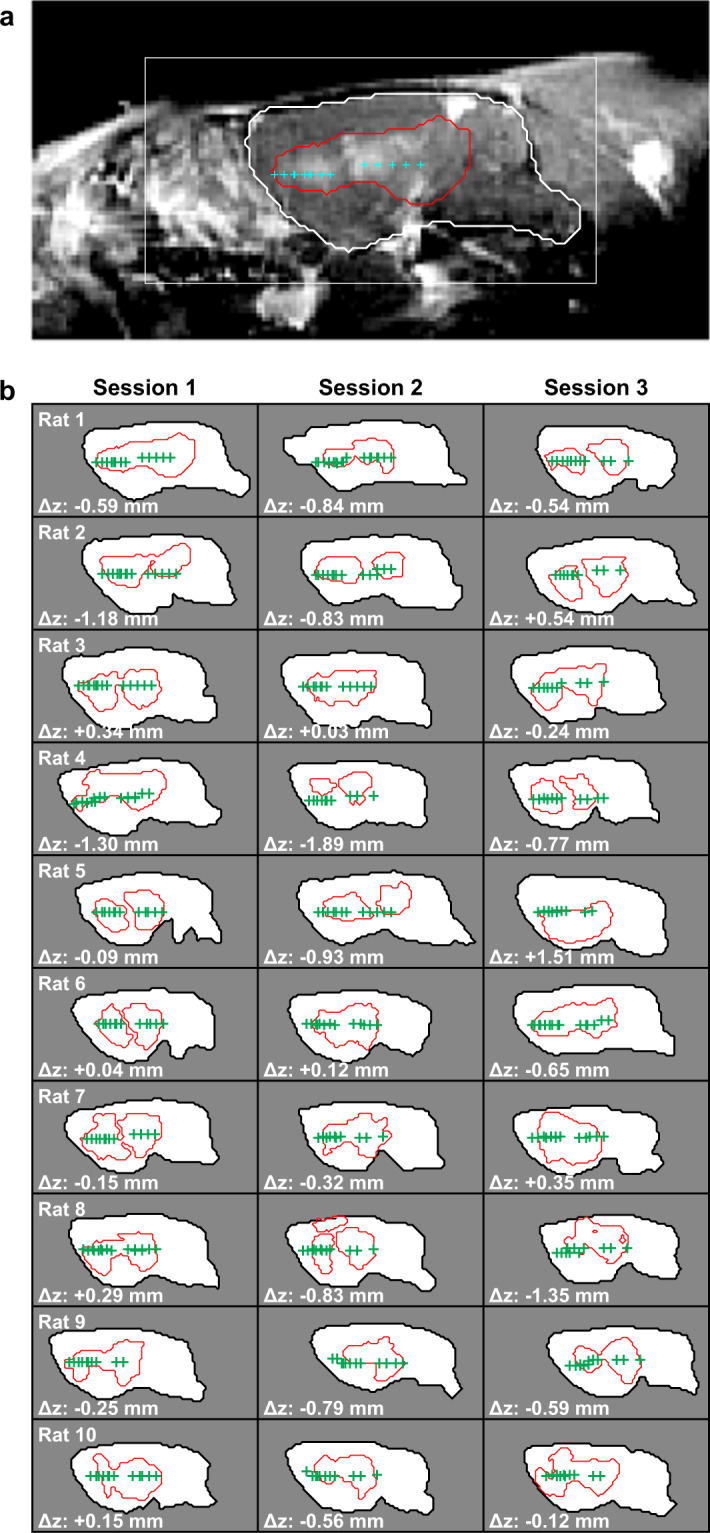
(a) Sagittal contrast-enhanced T1-weighted MRI showing BBB disruption, evident in the area outlined in red. The targets that were within ±1 mm of this image plane are shown, and the brain is segmented with a white contour. (b) Segmentations of the brain and the area with evident BBB disruption for 30 consecutive sessions. The targets are indicated. In most cases, the extent of the BBB disruption was contained within the brain. In a few cases, the disruption reached the brain surface proximal to the transducer. The distance between the average depth of the segmented BBB disruption and the target plane was less than one millimeter in 25/30 sessions.
